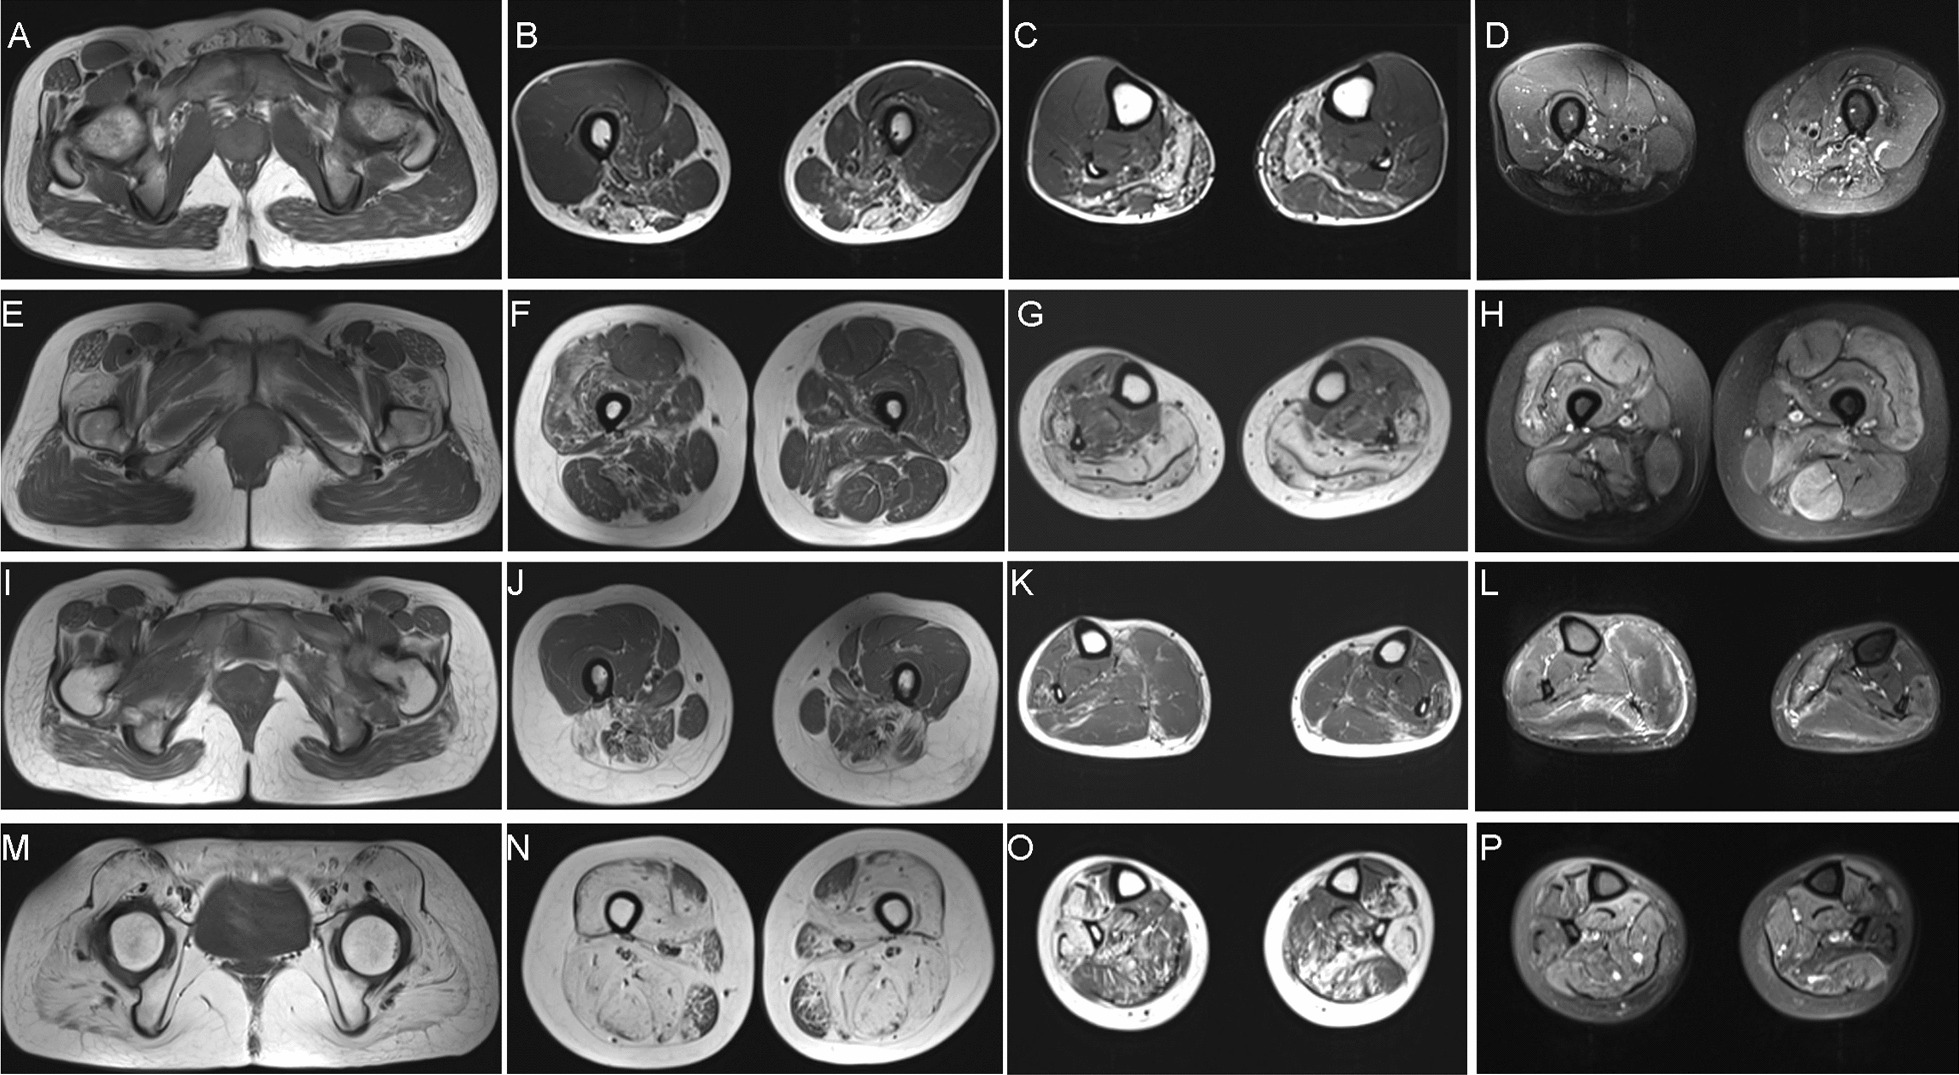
Representative T1-weighted images (A–C, E–G, I–K, M–O) and fat-suppressed T2-weighted MRI images (D, H, L, P) of LGMD-R1-calpain3-related, dysferlinopathy, LGMD-R7-telethonin-related, and LGMD-R9-*FKRP*-related (A–D). Muscle MRI of LGMD-R1-calpain3-related revealed severe involvement of the AM and posterior compartment muscles (including the SM, ST and BF at the thigh level and the GC and SO at the calf level), GR hypertrophy and a normal appearance of the RF, QF and S at the thigh level and of the TA, TP and EDL at the calf level. E–H Muscle MRI of a patient with dysferlinopathy indicated that the posterior and anterior compartments of the thigh muscles were equally involved and that fatty infiltration of the GC, SO and PL was severe. Compared with LGMD-R1-calpain3-related, the posterior compartment of the thigh muscles was less affected, but edema of the QF and ST was predominantly observed in dysferlinopathy. I–L LGMD-R7-telethonin-related, showed severe involvement of the GMa, hamstrings, BL and AM, but the QF was relatively preserved; marked and asymmetric edema was present in the GC, SO and TA. M–P In LGMD-R9-*FKRP*-related, the anterior and posterior muscles in the thigh were involved; however, the S, GR and RF were relatively preserved. At the calf level, the involvement of the PL and EDL was more severe than that of the GC and SO, whereas the TA was relatively spared. Edema of the lower legs showed the opposite pattern, in which the TA, GC, SO, TP and FDL were involved, but the PL and EDL were unaffected. *AM*: adductor magnus; *BF*: biceps femoris; *EDL*: extensor digitorum longus; *FDL*: flexor digitorum longus; *GC*: gastrocnemius; *GMa*: gluteus maximus; *GR*: gracilis; *PL*: peroneus longus; *RF*: rectus femoris; *QF*: quadriceps femoris; *S*: sartorius; *SO*: soleus; *ST*: semitendinosus; *SM*: semimembranosus; *TA*: tibialis anterior; *TP*: tibialis posterior)
